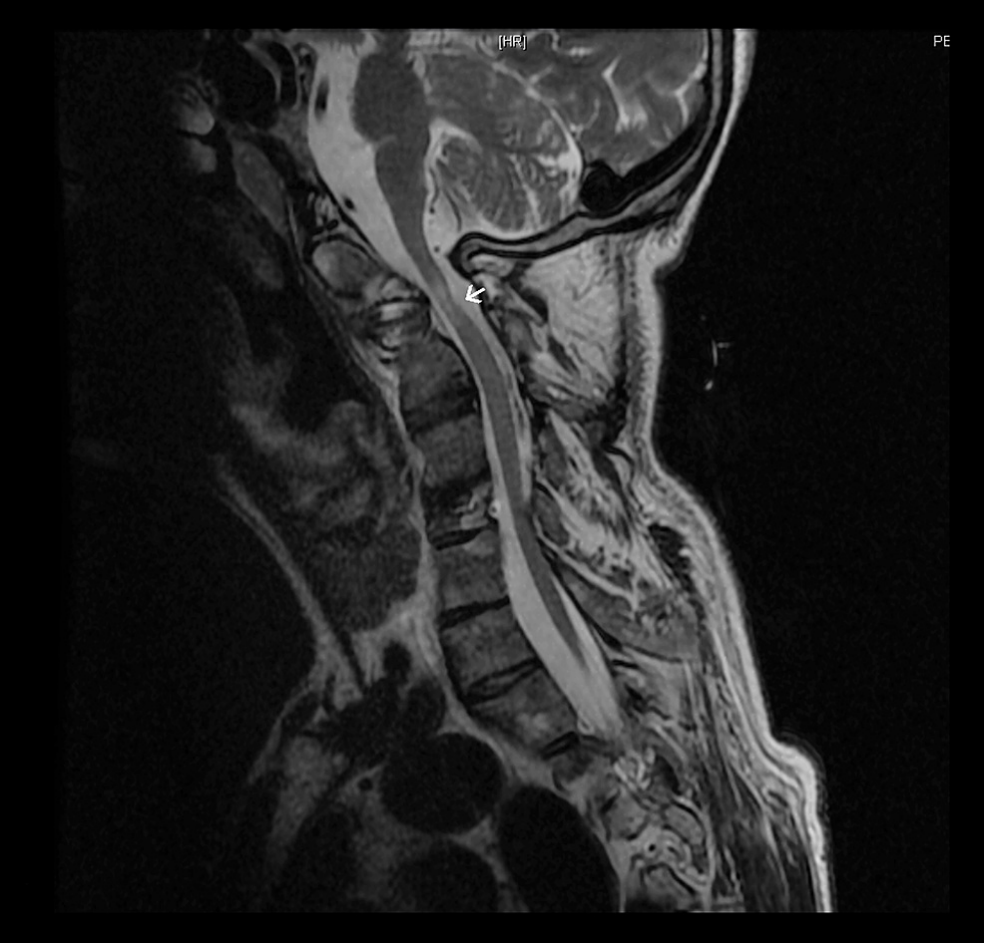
Cervical MRI, sagittal plane, T2-weighted image Spinal canal stenosis is visible at C1-C2 level, associated with spinal cord hyperintensity (arrow).
